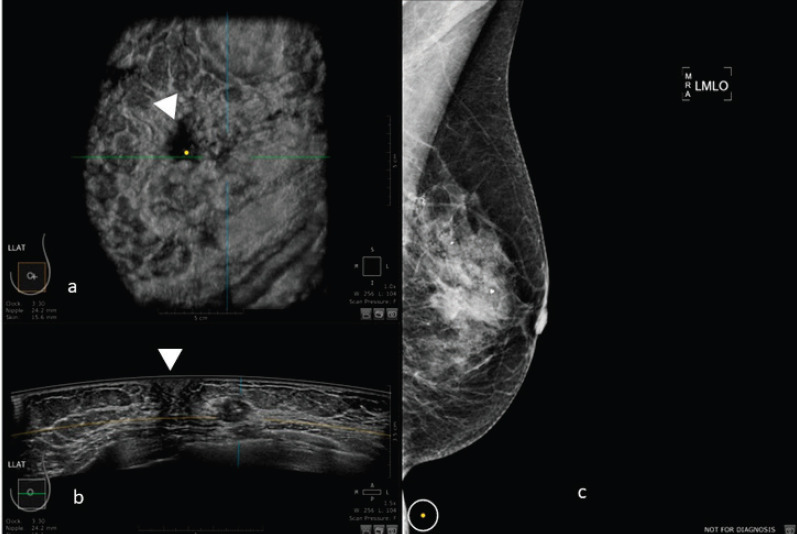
50-year-old patient with biopsy proven left breast carcinoma. a: 3D ultrasound (ABUS) image at the dedicated workstation. Reconstructed coronal plane: the lesion is marked as a reference point. The nipple is marked with the arrowhead. b: 3D ultrasound (ABUS) image at the dedicated workstation. Reconstructed axial plane: the lesion is marked as a reference point. The nipple is marked with the arrowhead. c: Full field digital mammography of the left breast in which the lesion is fairly visible.
